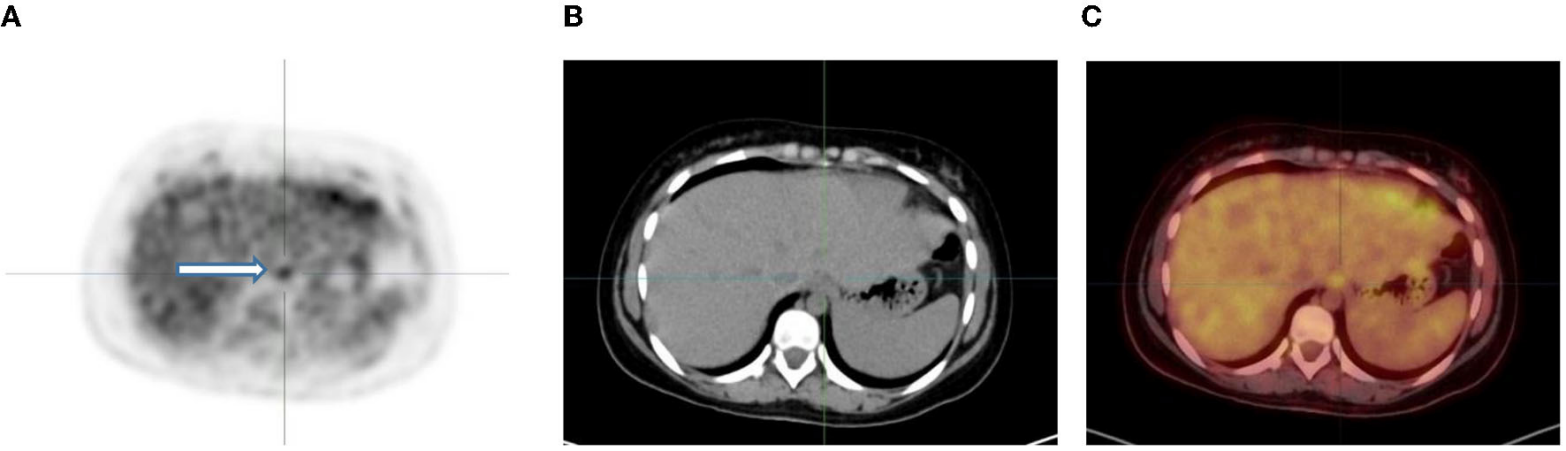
6-year-old female with Ewing's sarcoma of the left proximal tibia. Axial PET (A), CT (B) and fused PET/CT (C) showing mild diffuse uptake in the gastroesophageal junction (white arrow and crosshairs) that is physiological.
